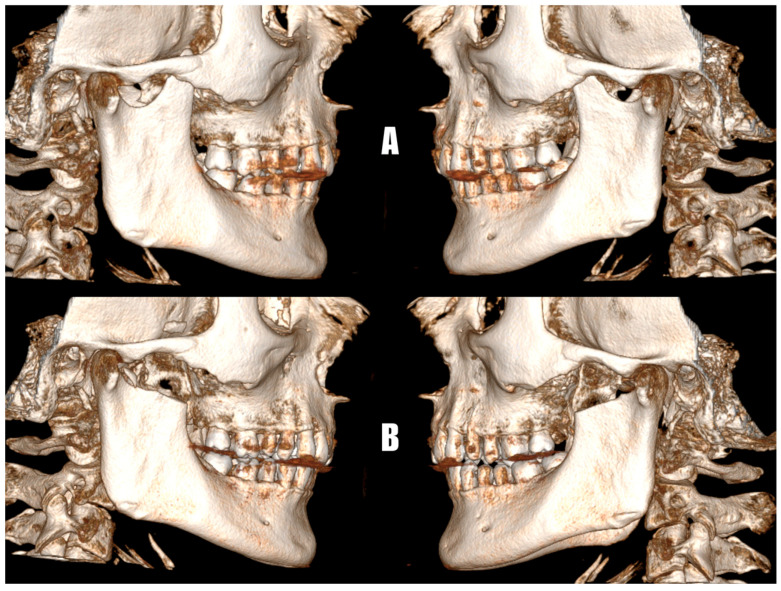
Three-dimensional reconstruction of the patient’s CBCT scans showing the coronoid processes before (A) and after (B) surgical resection.
